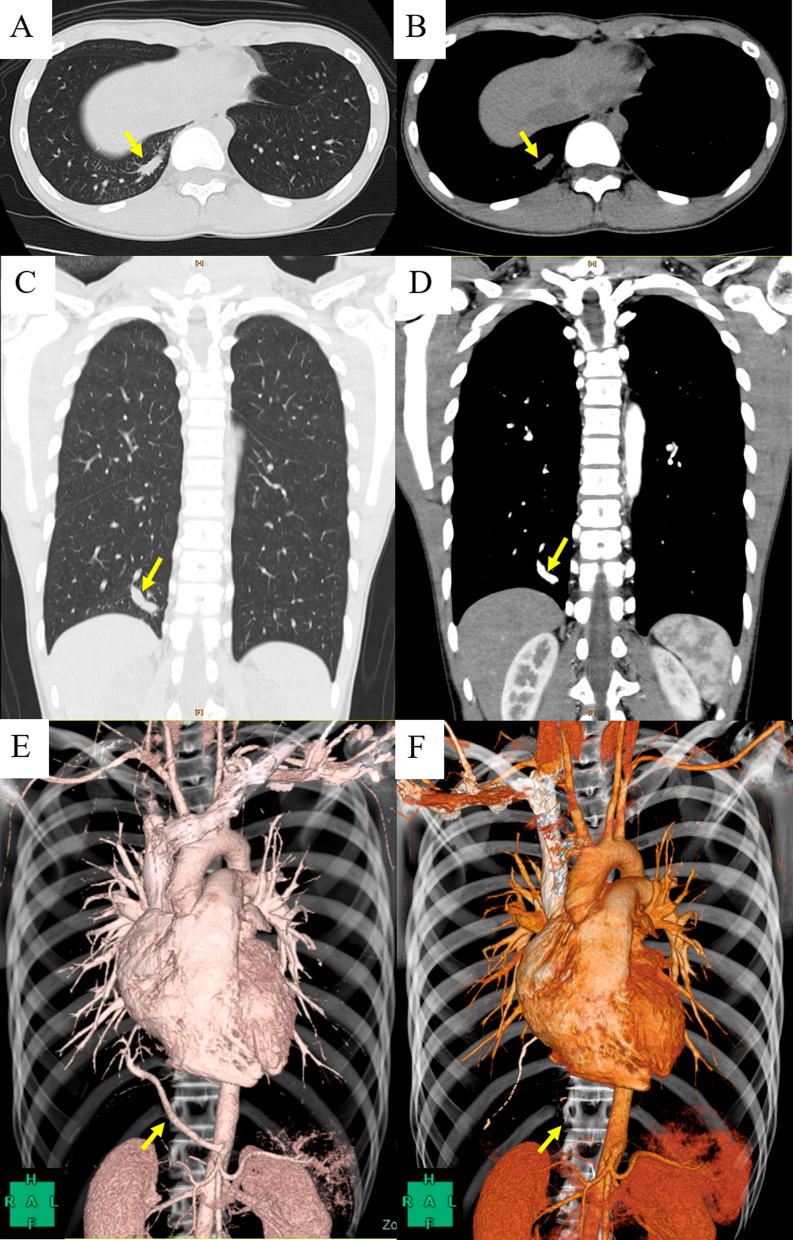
Non-contrasted axial (a, b) and coronal (c) computed tomography (CT) images; d contrast-enhanced coronal CT image; and e three-dimensional (3D) angiography showed the aberrant artery from the celiac artery (yellow arrow) flowing into the right dorsobasal segment (S10). f The findings of 3D angiography at the 3-month follow-examination showed that the remnants of the anomalous artery had become cord-like, without aneurysmal formation
